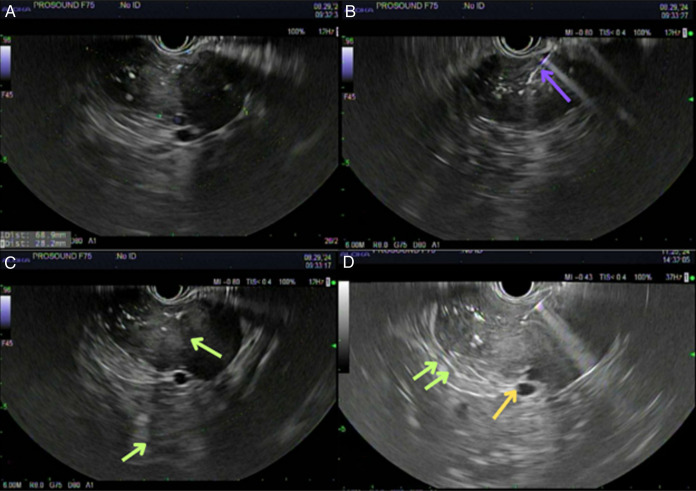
First EUS demonstrating a 68 × 28-mm pancreatic cyst that is anechoic/hypoechoic (A), with purple arrow pointing to fine-needle aspiration biopsy needle (B), and shadowing thought to be small calcium deposits demonstrated by green arrows (C). Second EUS demonstrating same green arrow shadowing as well as abutment of splenic vessels as shown as yellow arrow. EUS, endoscopic ultrasound.
